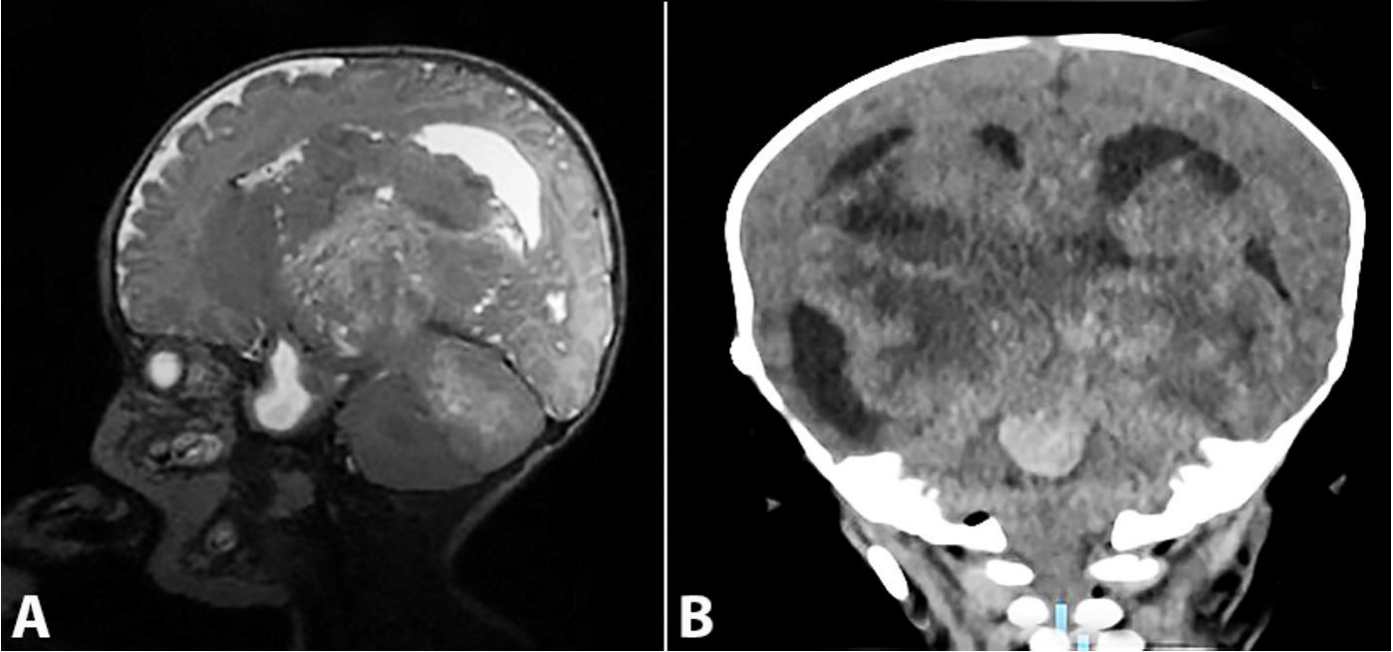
A – Magnetic resonance imaging, sagittal section: T2 sequence shows a heterogeneous cystic mass causing important dilatation of the ventricle system; B – Computed tomography of the coronal section, parenchymal (brain) window: shows a heterogeneous cystic mass causing important dilatation of the ventricle system.
